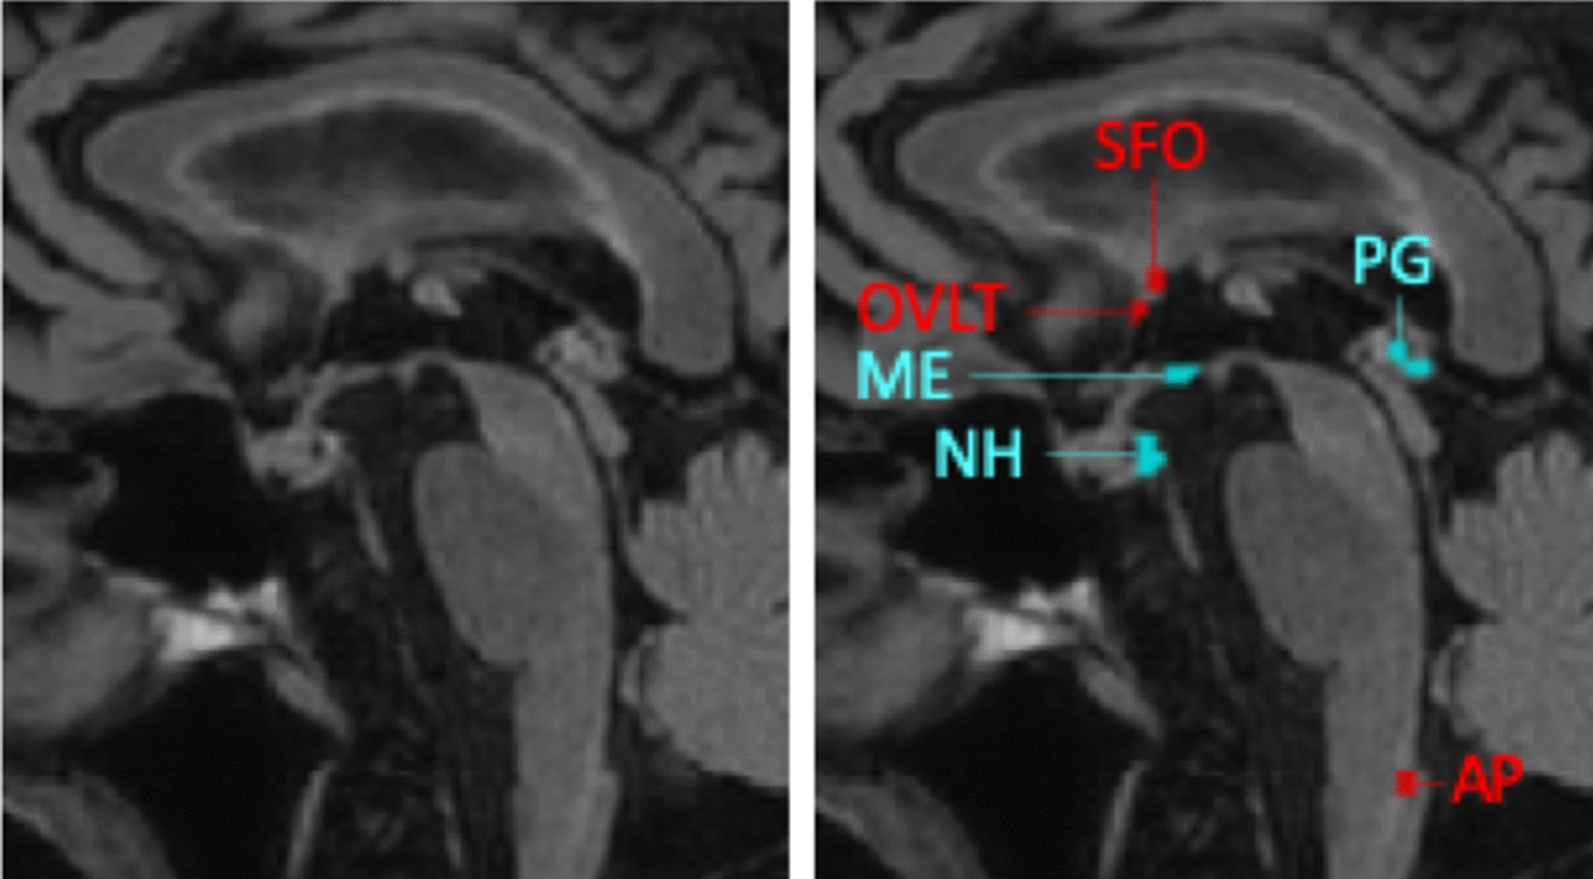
Example of a mid-sagittal anatomical FLAIR image and the regions-of-interest of the secretory (blue) and sensory (red) circumventricular organs. *NH* neurohypophysis, *ME*  median eminence, *PG* pineal gland, *SFO* subfornical organ, *OVLT* organum vasculosum of the lamina terminalis, *AP* area postrema
